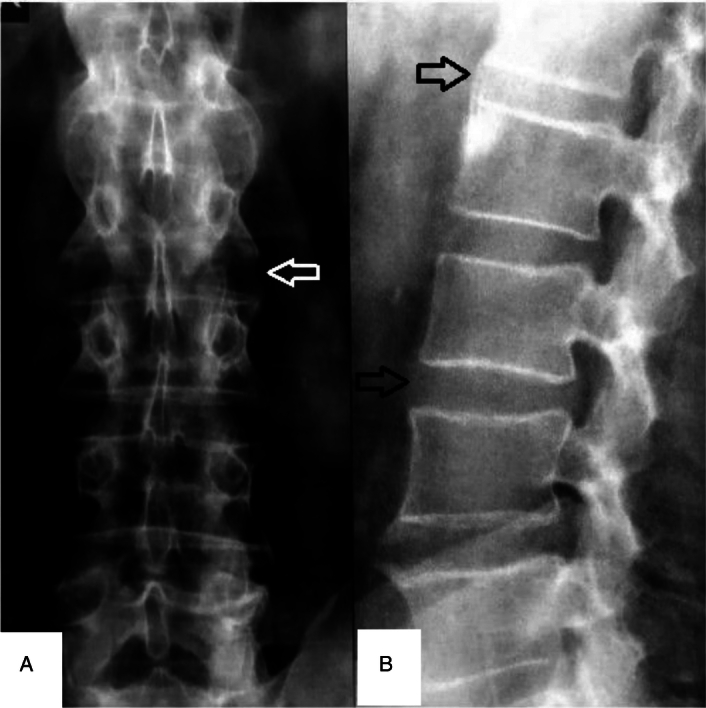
Spinal X-ray demonstrated bilateral grade II sacroiliitis and loss of lumbar lordosis (A, B).
